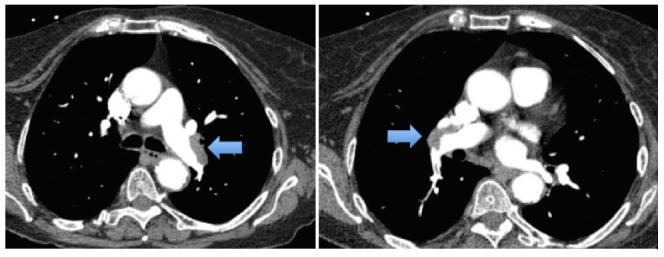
CT-chest: thrombi in the distal main right and left pulmonary arteries (arrows)
